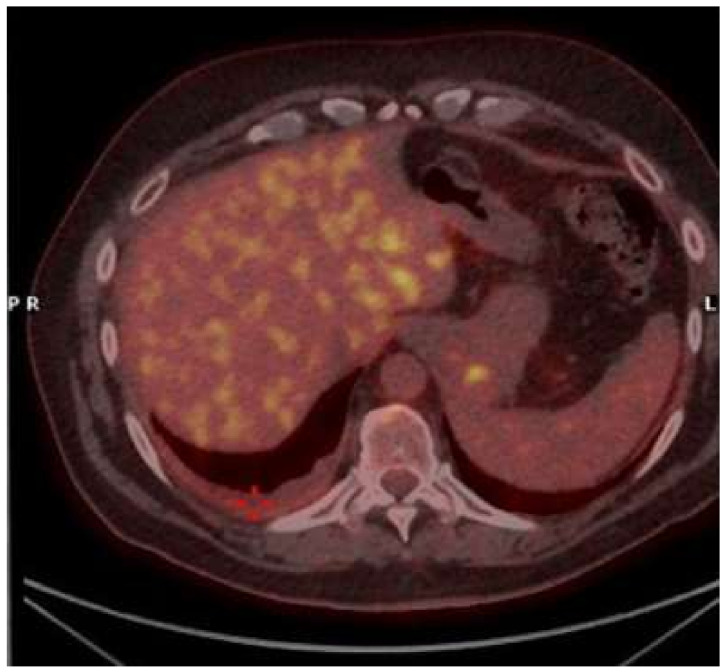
Positron emission tomography-fluorodeoxyglucose (PET-FDG) showed small amount of right pleural effusion associated with diffuse pleural thickening, resulted as slightly absorbing (red cross).
